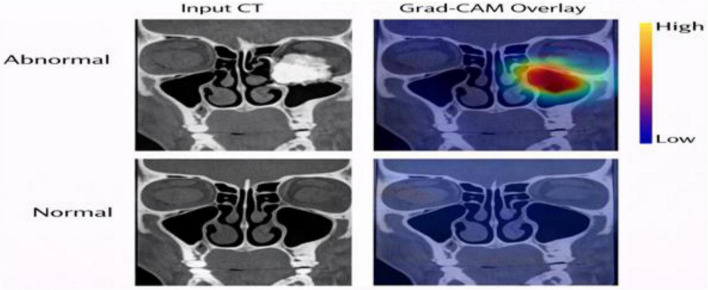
Grad-CAM activation patterns for representative sinus CT cases showing model attention over anatomically relevant regions.
